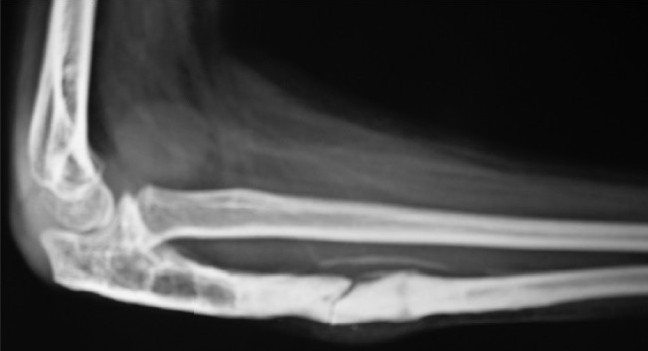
Lateral radiograph of elbow at final follow-up of 24 months. She had no symptoms and the graft has healed well. The radial head is well maintained
